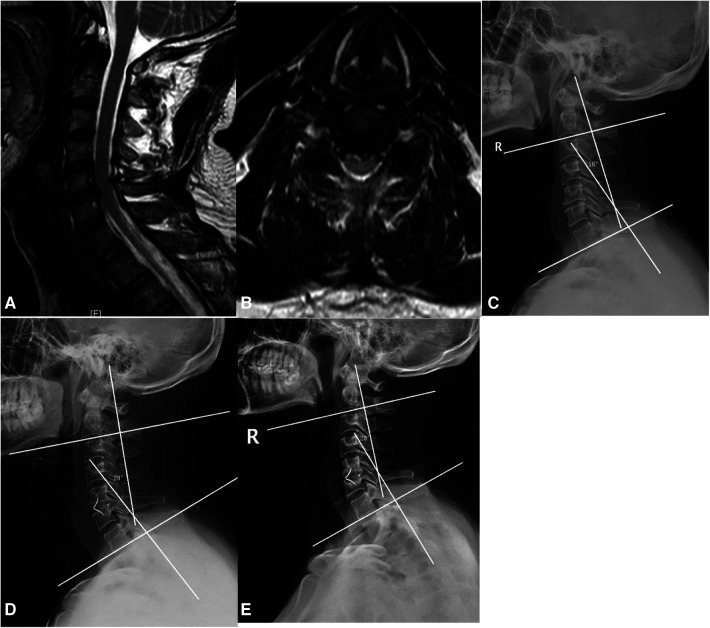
ACDF using ROI-C. (A) Sagittal MRI. (B) Axial MRI. (C) C2−7 cobb angle was 16°. (D) Immediately after surgery, C2−7 cobb angle was 29°. (E) C2−7 cobb angle decreased to 20° at the last follow-up visit.
